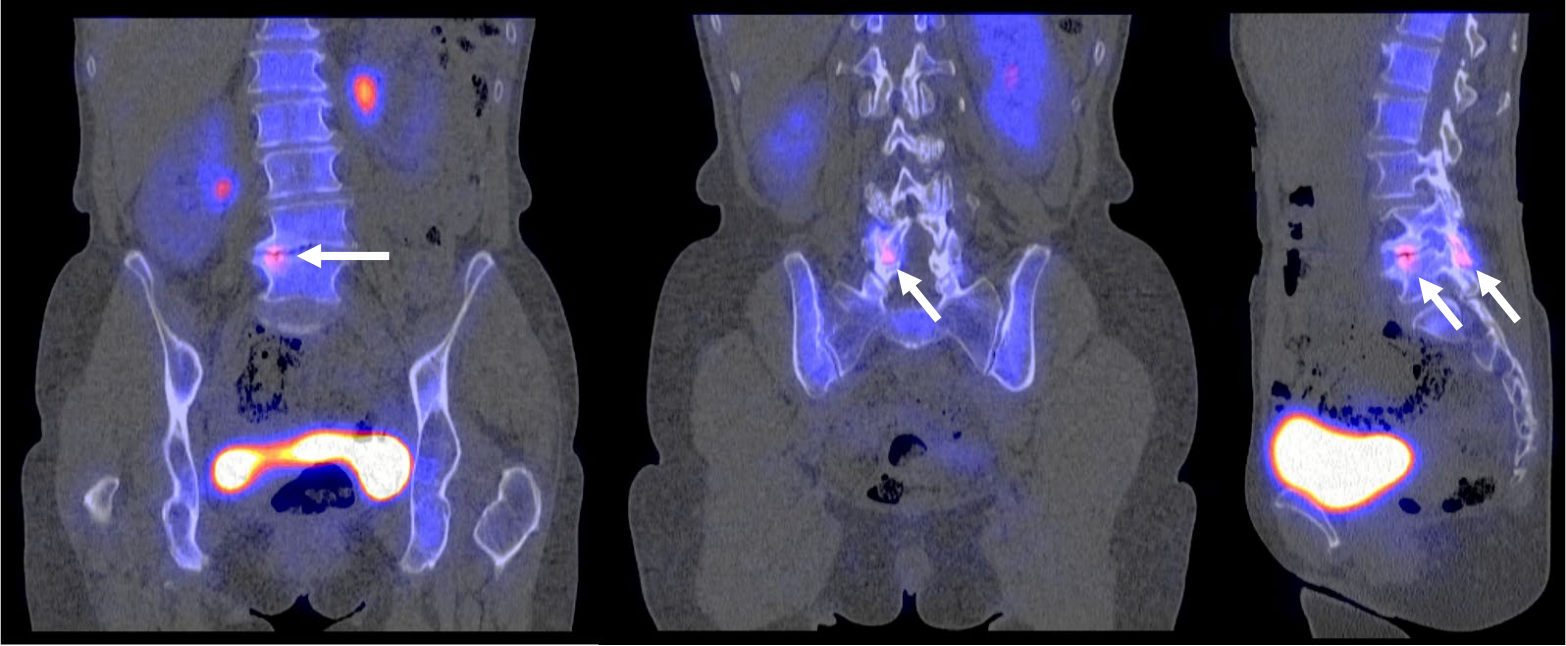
SPECT/CT of an FBSS patient using Tc-99m HDP to investigate the cause of persistent pain after left-sided laminectomy at level L5–S1. Focal radiotracer accumulation was observed at level L4–L5, both near the intervertebral disk and the right zygapophyseal joint. Tracer accumulation was considered to reflect active osteoarthritic changes. No reoperation was carried out
